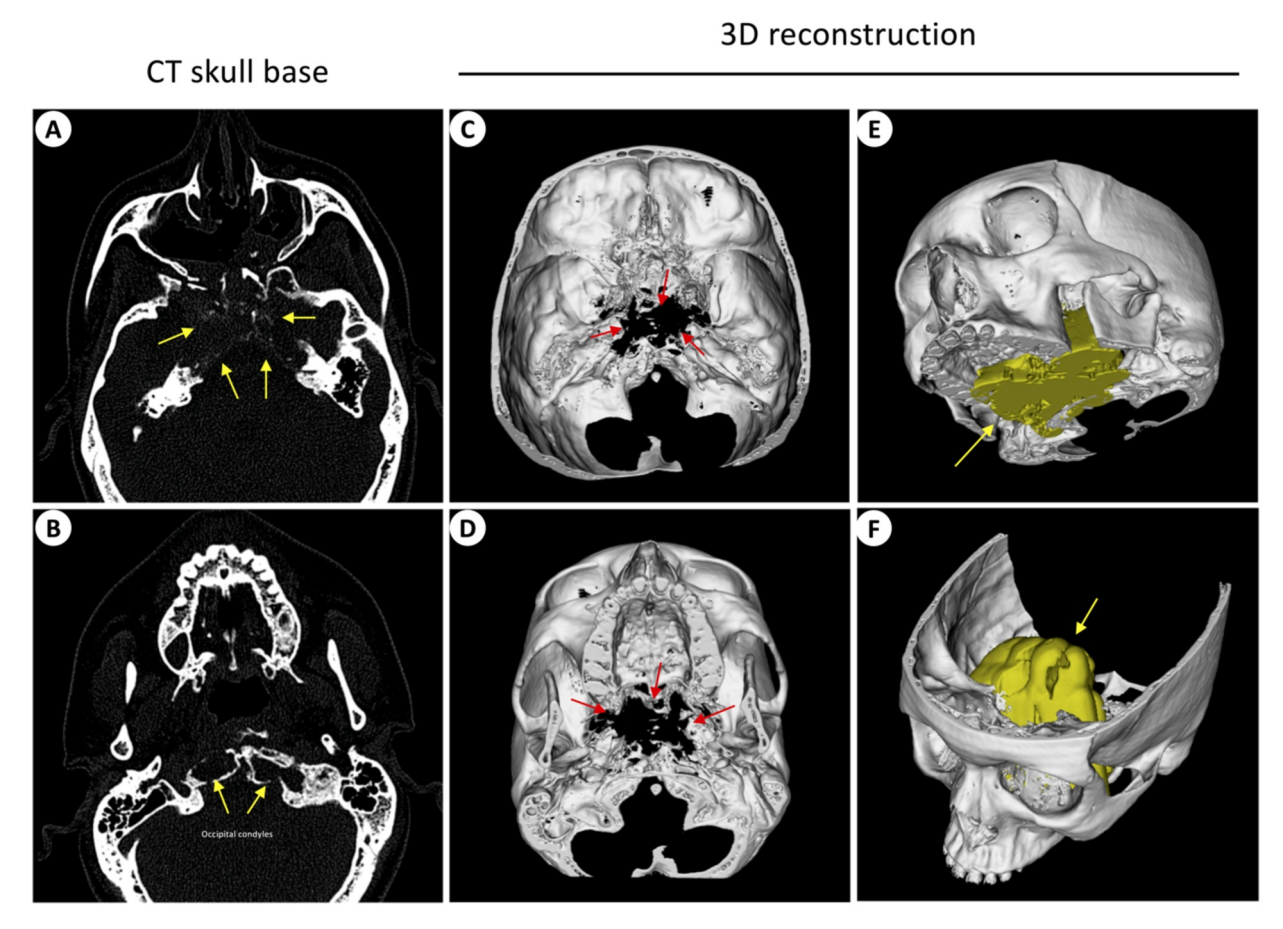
Skull CT Panels A and B show a large bony defect in the middle skull base (yellow arrows) extending inferiorly to the anterior aspect of the foramen magnum. Computerized reconstruction of the skull (C & D) shows the extent of the defect (red arrows), and when co-registered with the 3D-rendered tumour from the contemporaneous MRI scan (E & F, yellow arrows) confirms a near-total replacement of the middle skull base with large tumour tissue
